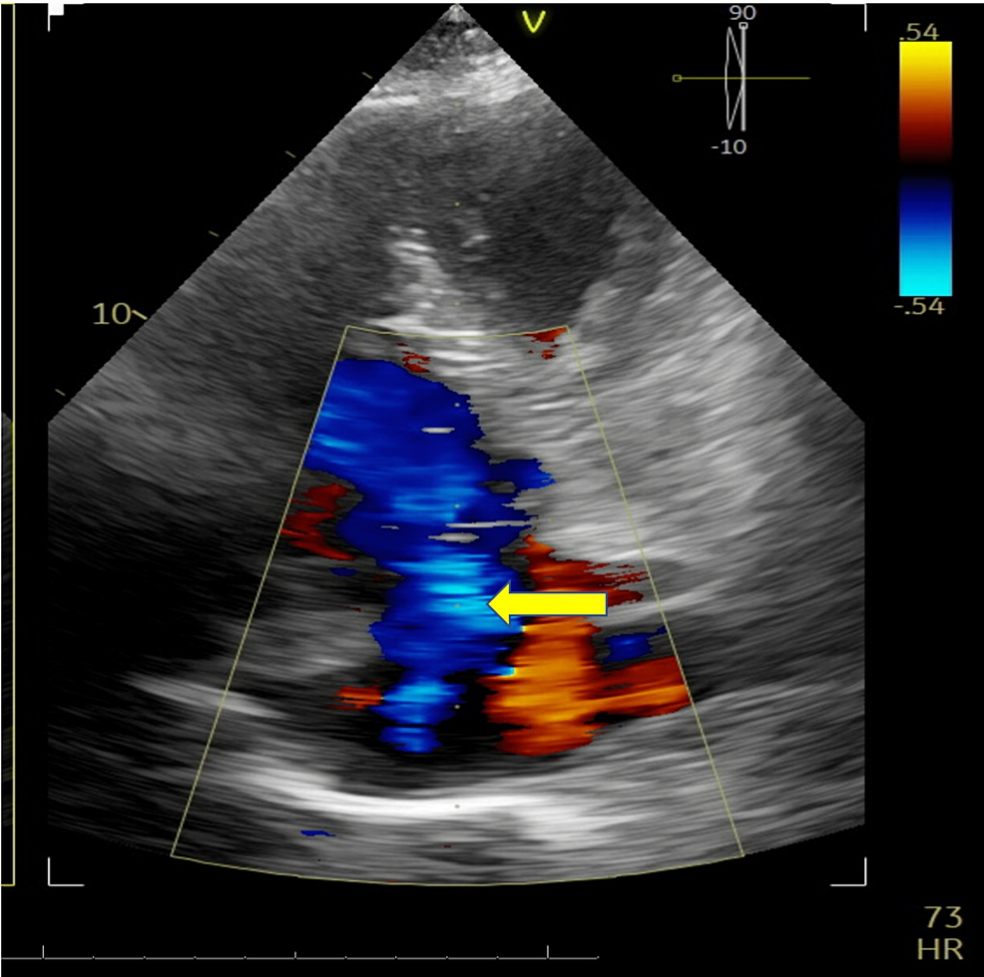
TTE with color Doppler demonstrating severe tricuspid regurgitation. The yellow arrow highlights regurgitant flow of blood from the right ventricle into the right atrium during systole. TTE, transthoracic echocardiogram
